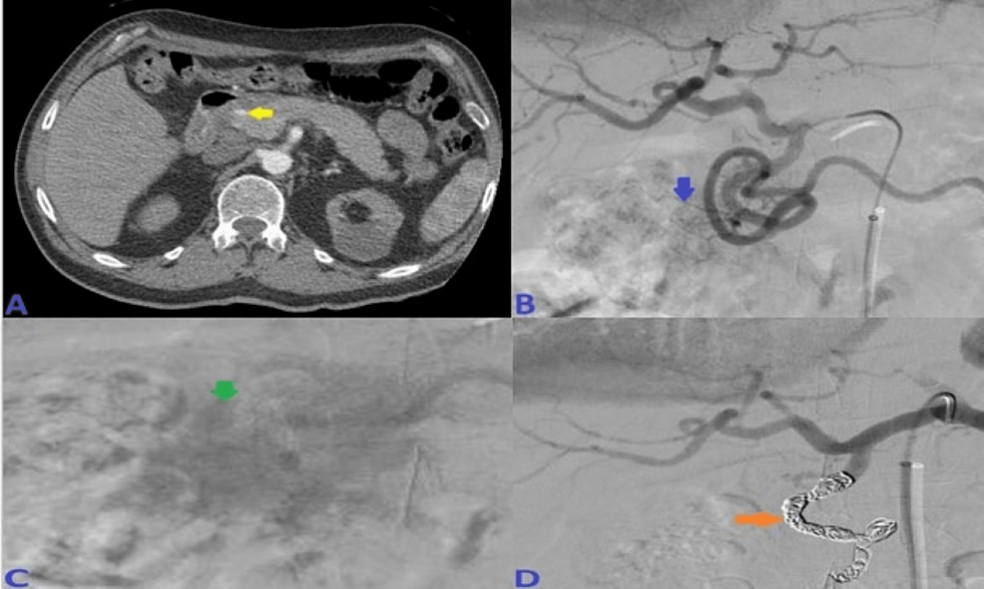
A: CT angiogram axial image of the abdomen demonstrated irregularly dilated and exposed mucosal duodenal arterioles corresponding to actively oozing crated duodenal ulcer in the duodenal bulb seen on prior endoscopy. B: Sequential selective vascular catheterization of celiac and GDA angiograms demonstrated duodenal bleeding territory with many irregularly dilated arteriolar feeders from branches of the GDA with focal blush as seen on delayed venous phase (image C). D: Post-embolization celiac angiogram demonstrated no evidence of active bleeding signs, and complete resolution of the above findings after 13 coils' deployment in “sandwiching” appropriate positions, beyond the takeoff of main GDA, with adequate hepatic arterial inflow at the end of the embolization. CT: computed tomography; GDA: gastroduodenal artery.
